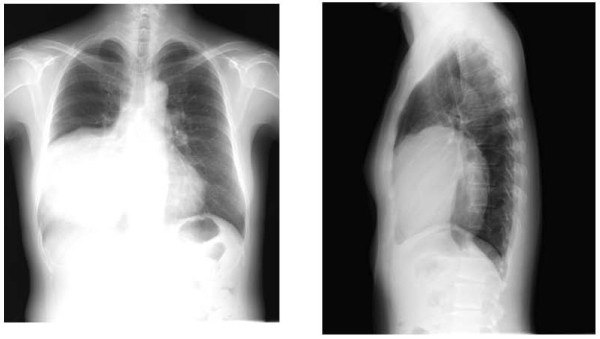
Plain chest radiograph showing a mass lesion in the right lower lung.
